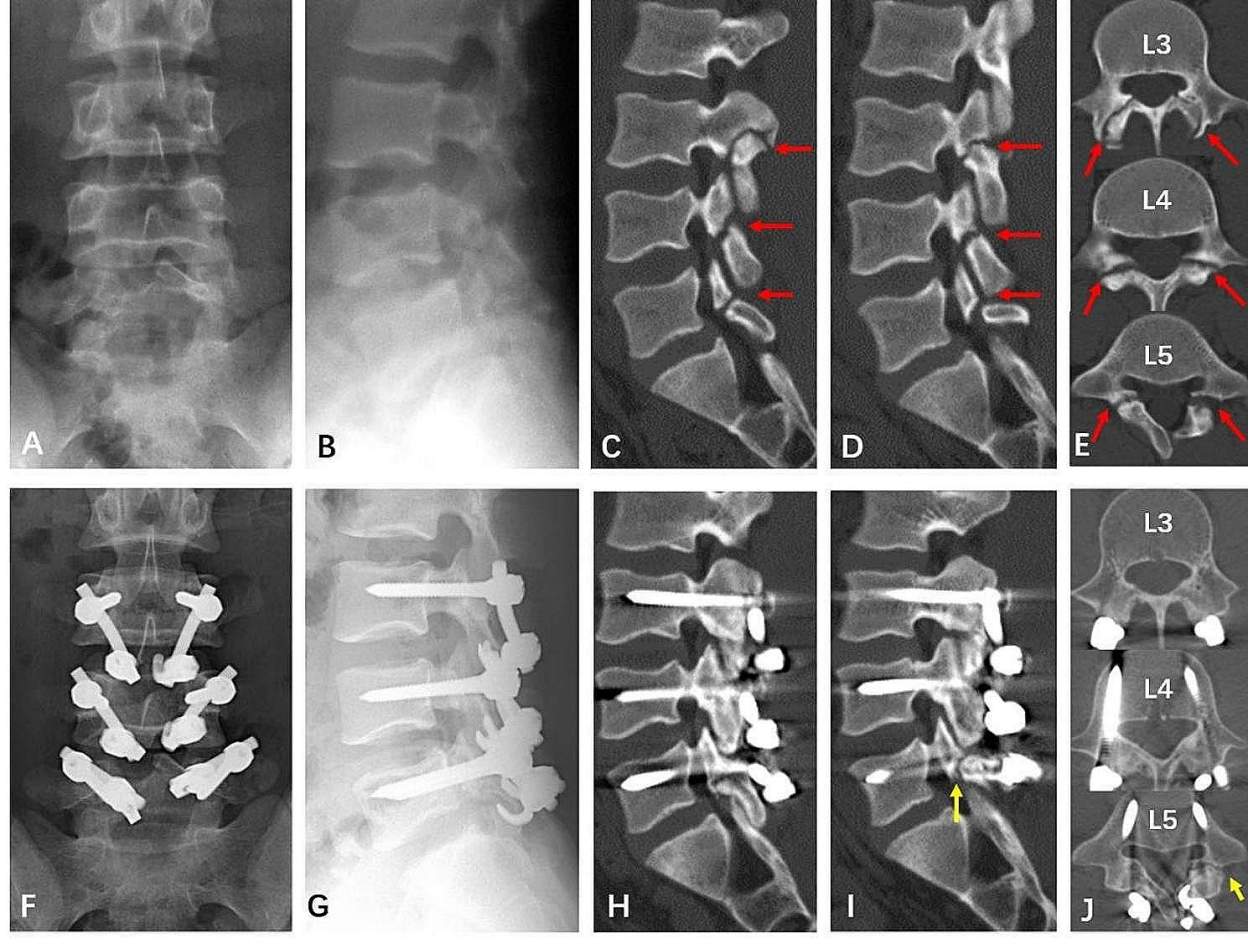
A16-year-old male, preoperative X-rays (A, B) and CT images (C, D, E) showed L3, L4 and L5 bilateral spondylolysis (red arrows in C, D and E) and L5 spina bifida occulta (E). Postoperative X-rays (F, G) and CT images (H, I, J) at two-year follow-up showed union of L3, L4 and right L5 spondylolysis and non-union of left L5 spondylolysis. He had a normal daily life and no low back pain
